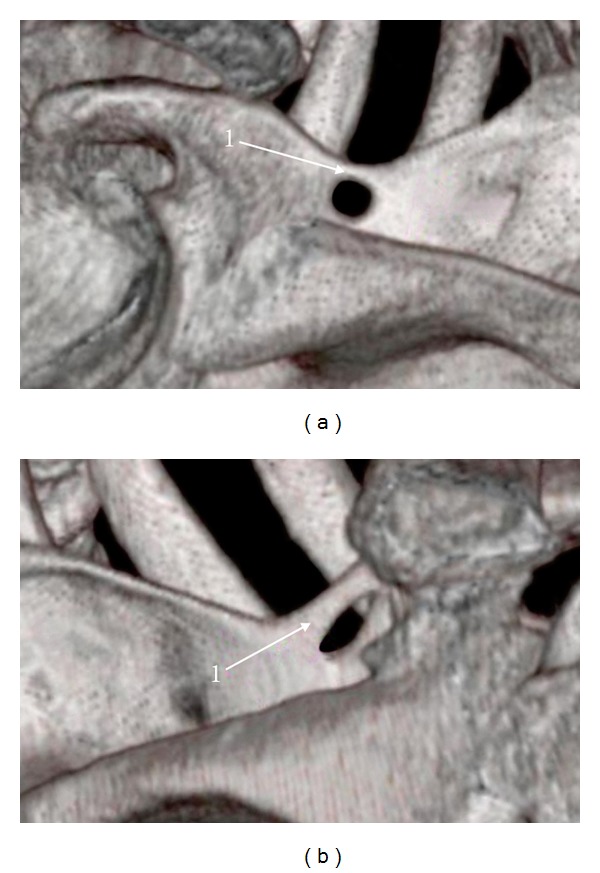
Three-dimensional volume rendering (VR) multidetector computed tomography (MDCT) of the scapula: 1: superior transverse scapular ligament (STSL). (a) Fan-shaped type of ossified STSL, (b) band-shaped type of ossified STSL.
